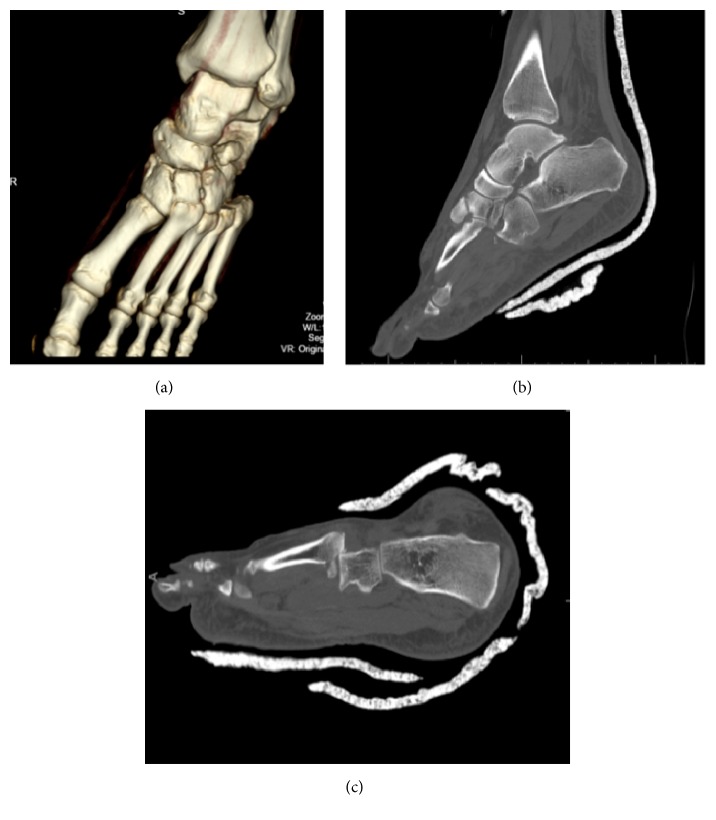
CT scan demonstrating dislocation of fourth and fifth tarsometatarsal joints.
